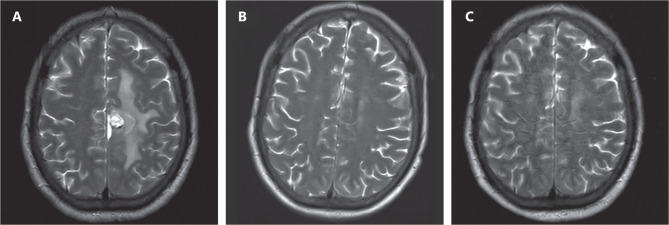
(A) MRI image of a patient with lymphoma in the right frontal and parietal area before treatment. (B) MRI showing marked tumor shrinkage after 2 cycles of treatment. (C) MRI showing tumor disappearance after 4 cycles of treatment. (According to our evaluation criteria, the size of the lesion was approximately 1.5 × 2.0 cm before chemotherapy; the size of the lesion was 0.9 × 0.8 cm after 2 cycles; the efficacy was evaluated as partial remission; the lesion disappeared after 4 cycles; and the efficacy was evaluated as complete remission.)
